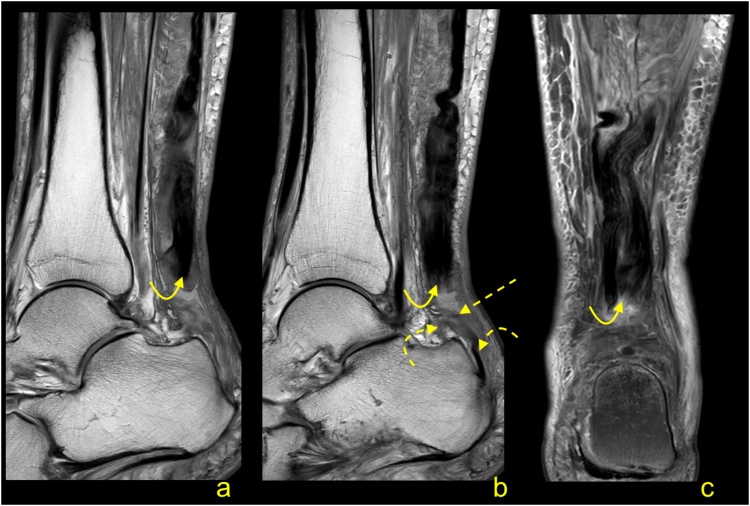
Total Achilles tendon rupture in the distal part 1 week ago (dashed arrow). MRI revealed that the proximal stump had retraction and shortening (curved arrow). The distal stump is frayed with volume reduction (curved dashed arrow). A and b- PD- weighted, c- T2- weighted with fat suppression.
